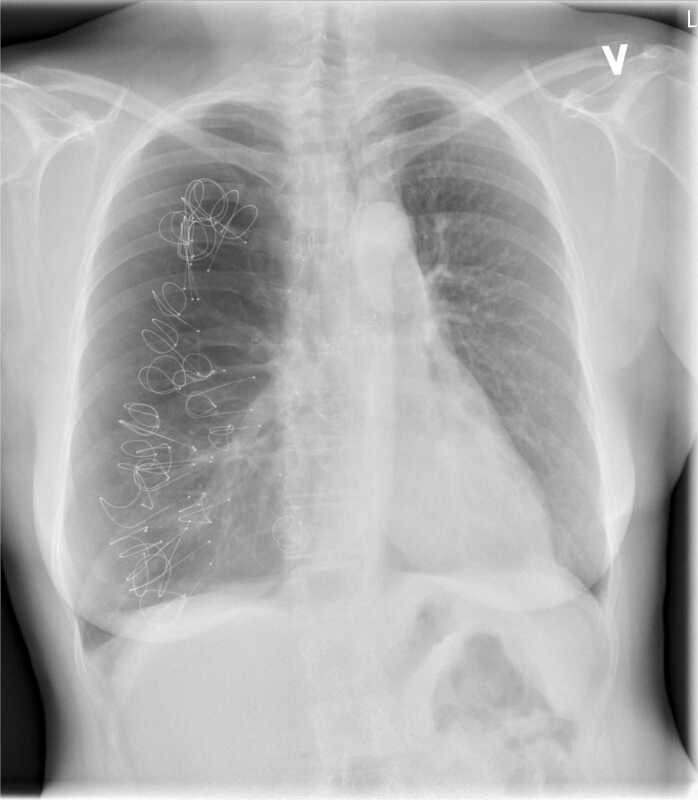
Chest X-ray after second LVRC treatment of right lower lobe. LVRC, lung volume reduction coils.
